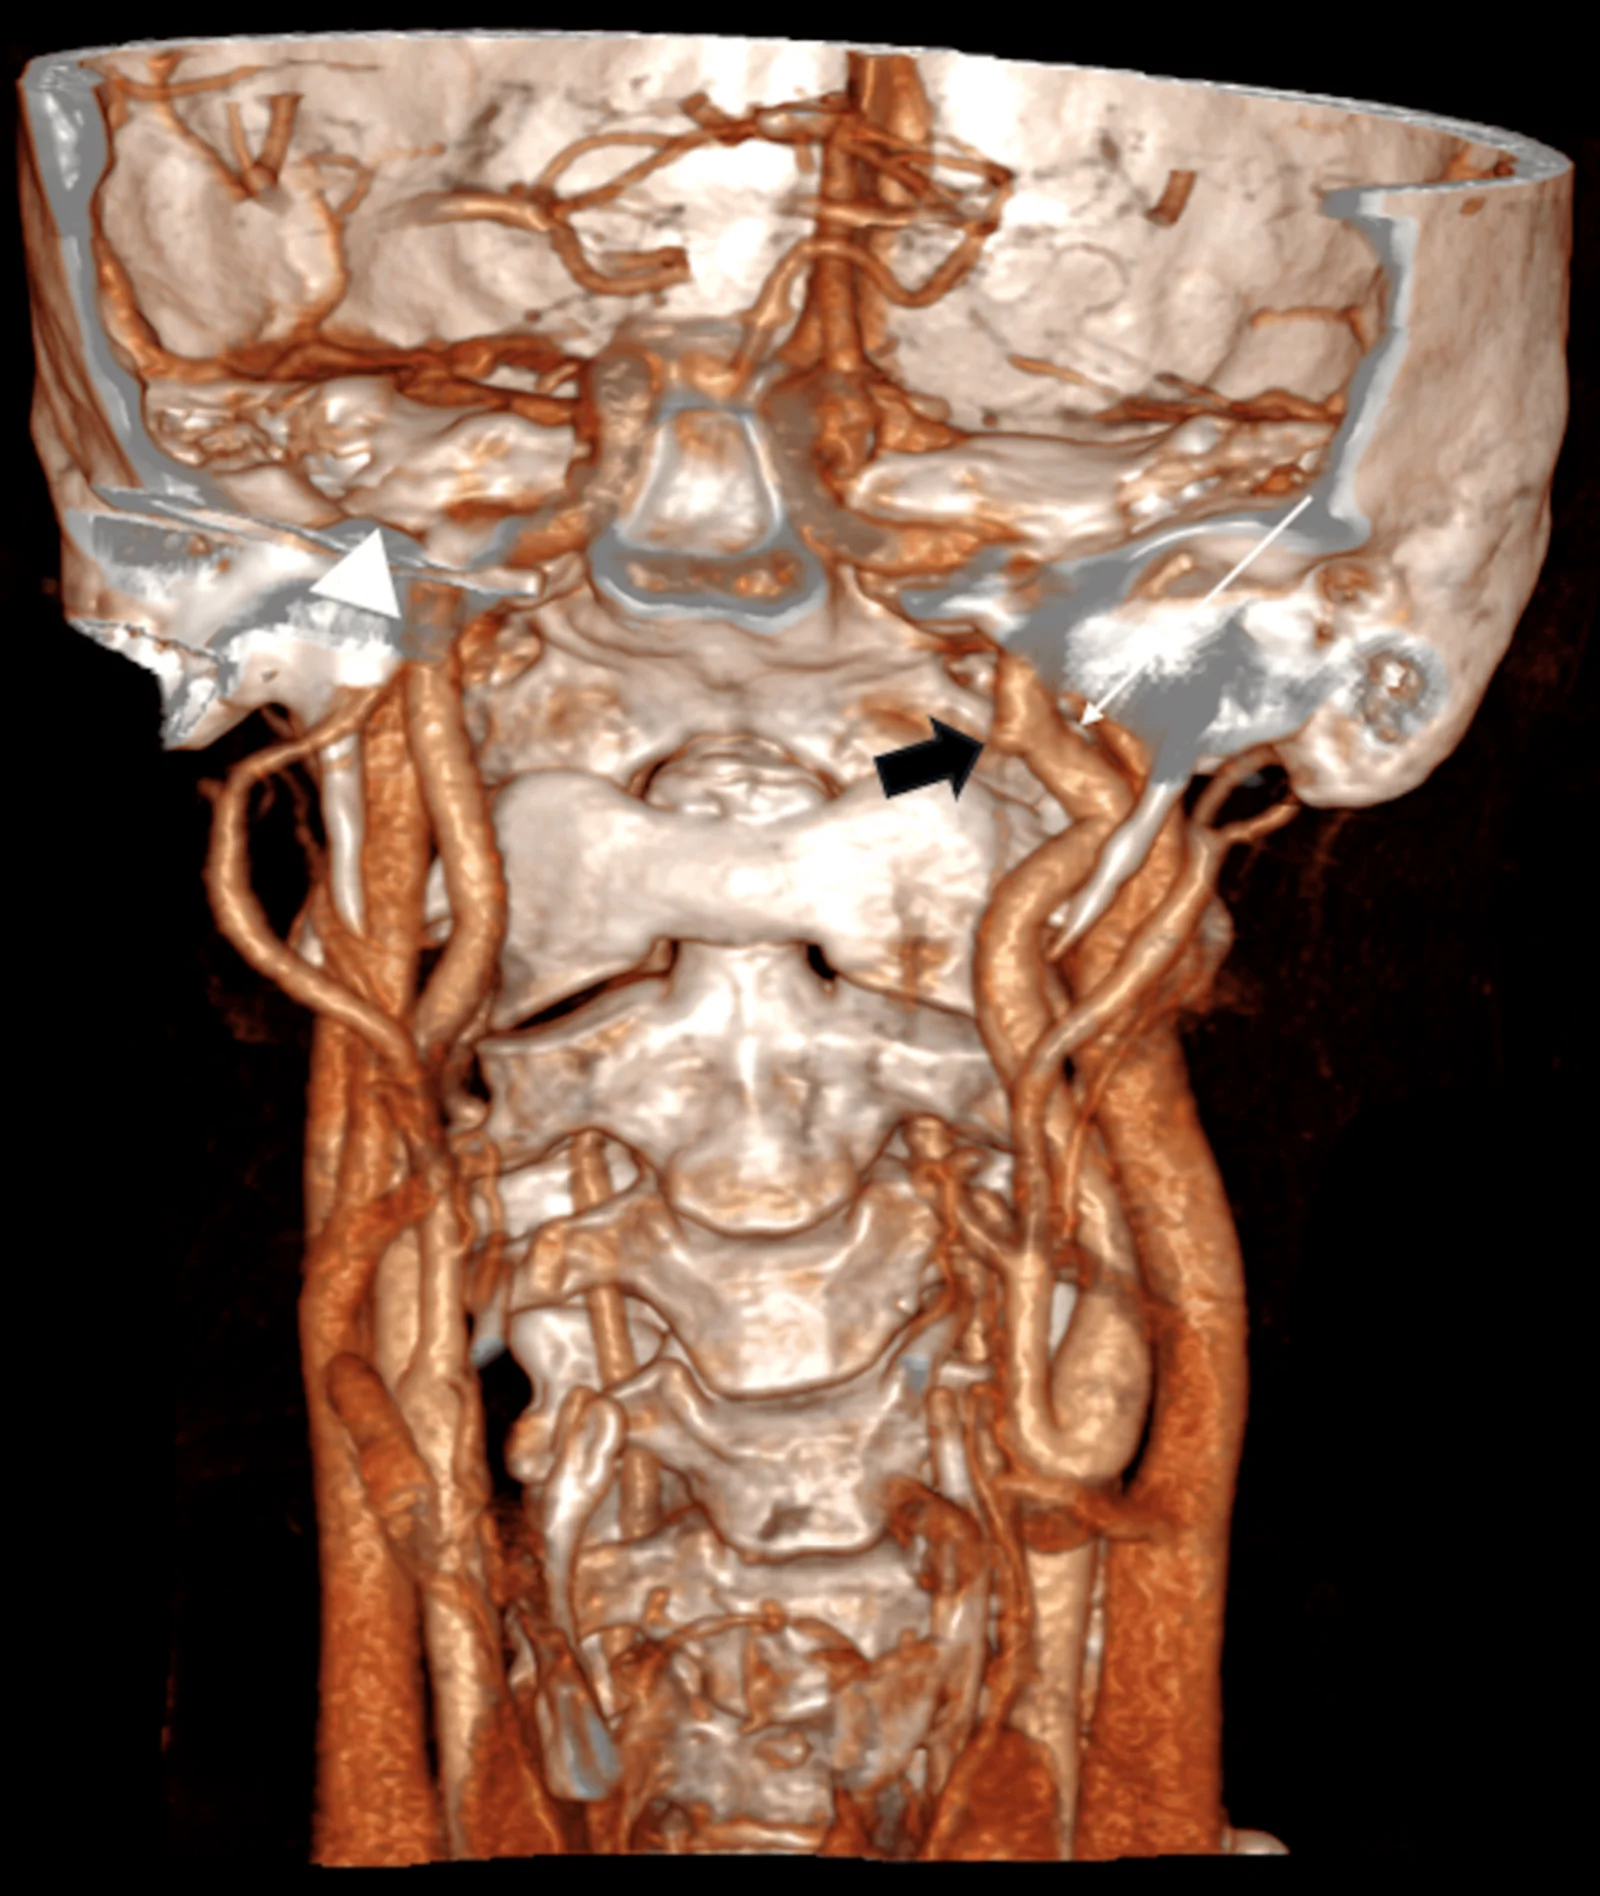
Surface-shaded CTA reconstruction demonstrating symmetric luminal diameter of the right (arrowhead) and left (white arrow) internal carotid arteries, with subtle vessel wall irregularity on the left secondary to dissection (black arrow) CTA: computed tomography angiography
